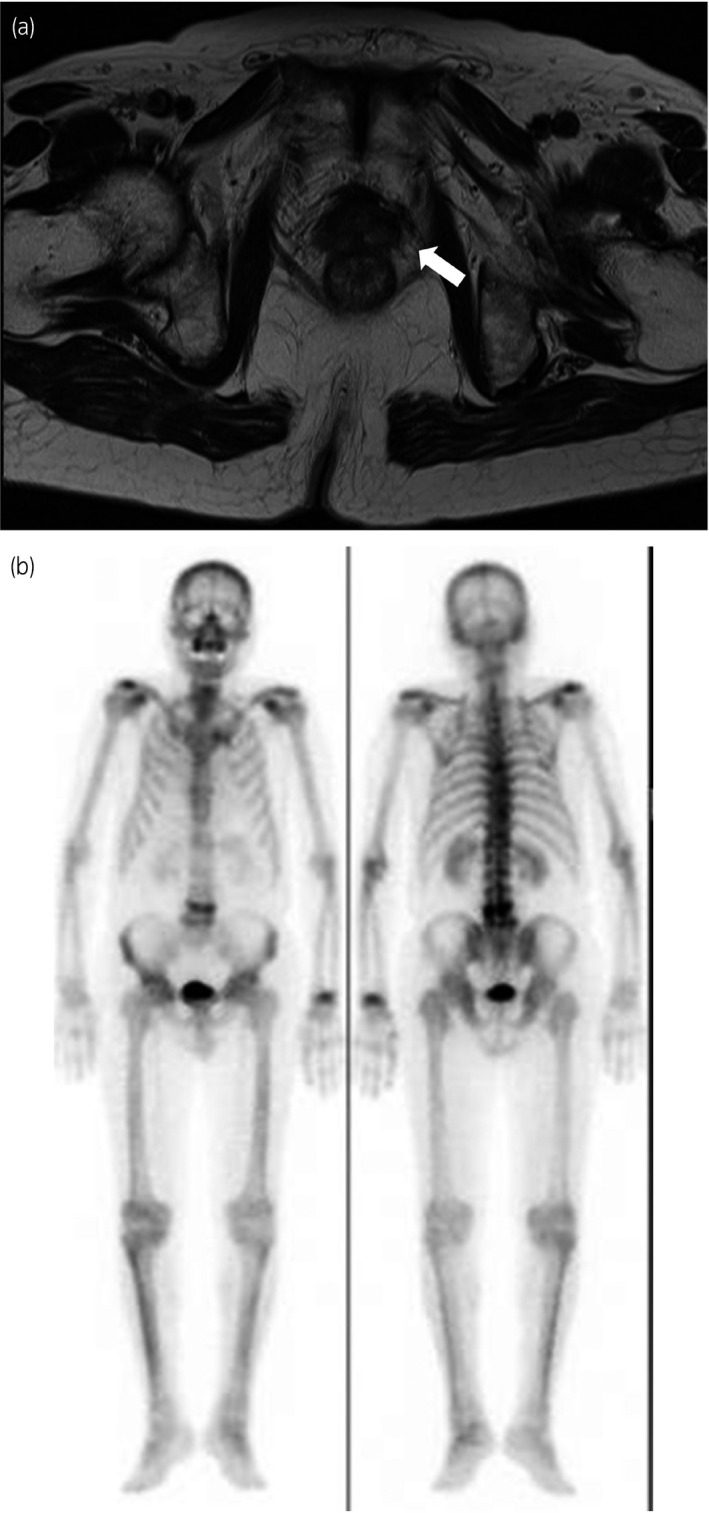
(a) Magnetic resonance image shows a clearly decreased prostate volume (arrows). (b) Bone scintigraphy image shows that the multiple bone metastases had disappeared.
